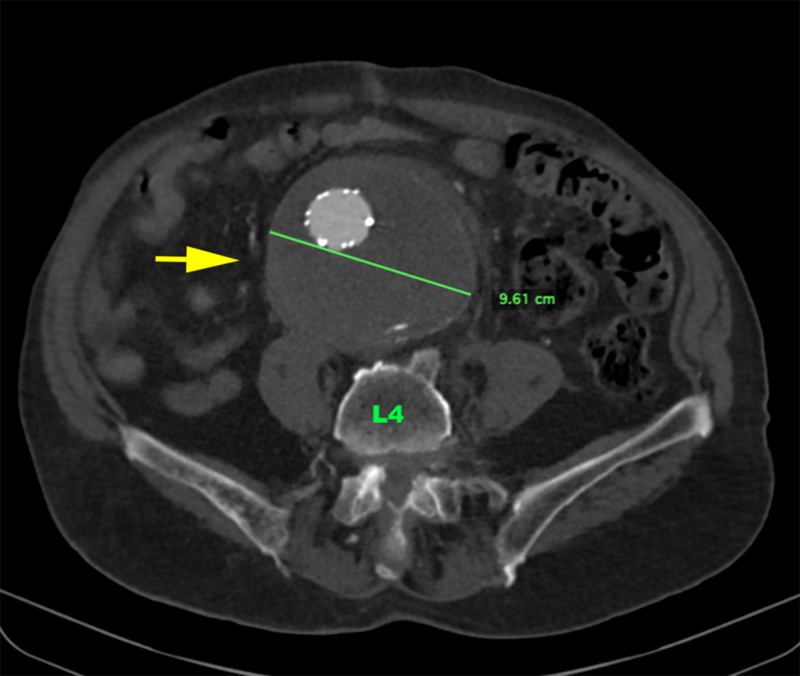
One-year follow-up CTA abdomen and pelvis axial image (level of L4) showing excluded AAA, which is stable in size at 9.6 cm (yellow arrow) AAA: abdominal aortic aneurysm; CTA: computed tomography angiography
